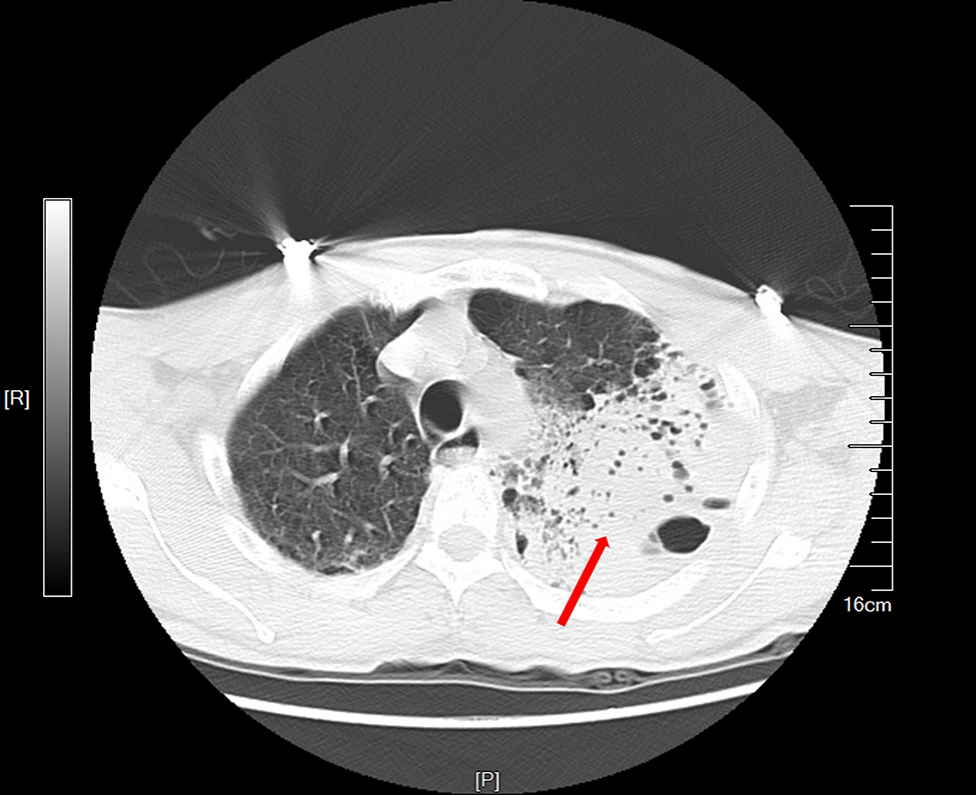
Chest computed tomography scan of patient who died of acute hypoxemic respiratory failure caused by *Burkholderia thailandensis*, China. Scan shows left lung consolidation and pleural effusion. Red arrow indicates a large high-density shadow in the left lung field. Scale indicates actual size of anatomical structures and lesions. P, posterior; R, right.
